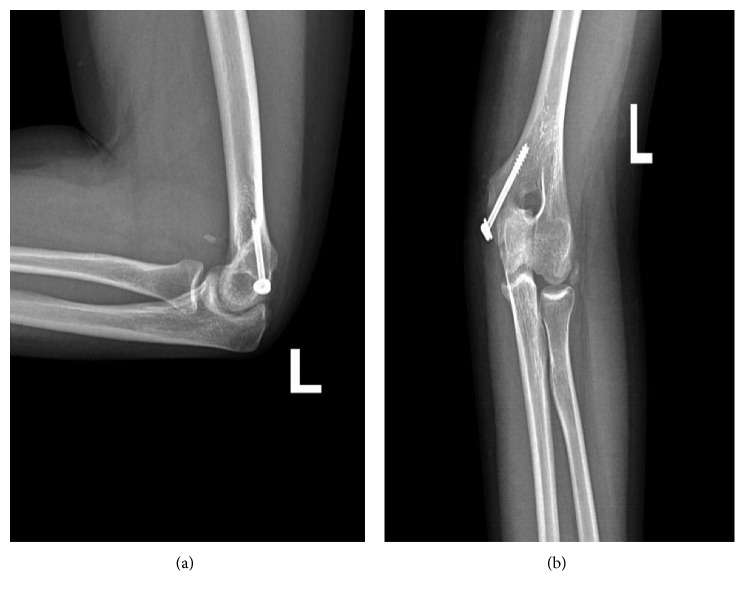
Lateral and anteroposterior radiographs of the left elbow joint 12 weeks postop showing union of the avulsed medial epicondyle fragment. Anterior and lateral ossifications seen indicate possible partial ligamentous injury that calcified with time and were not seen on previous radiographs.
